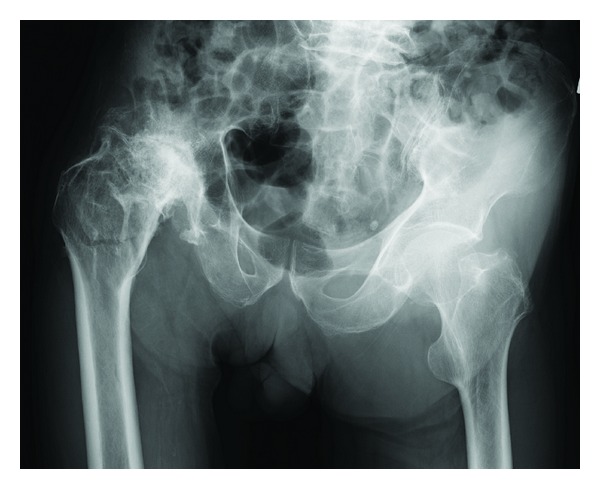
Anteroposterior radiograph showing an intertrochanteric fracture of the right hip joint that was ankylosed because of arthrodesis performed 56 years earlier.
